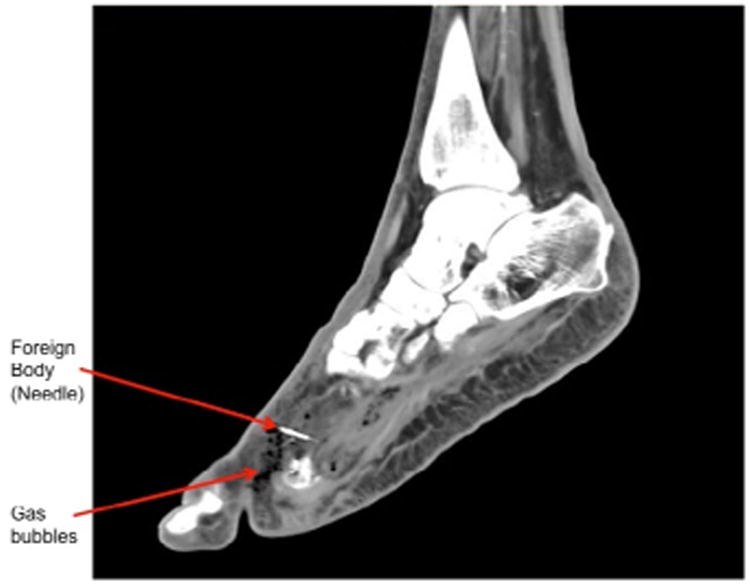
CT scan shows moderate air suggesting deep tissue infection with multiple tiny gas bubbles.
